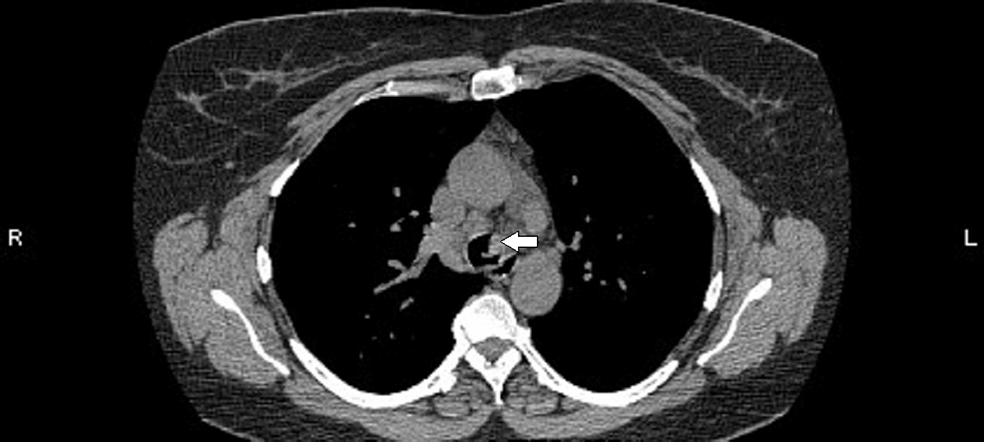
Axial CT scan with a white arrow demonstrating a possible squamous cell papilloma
